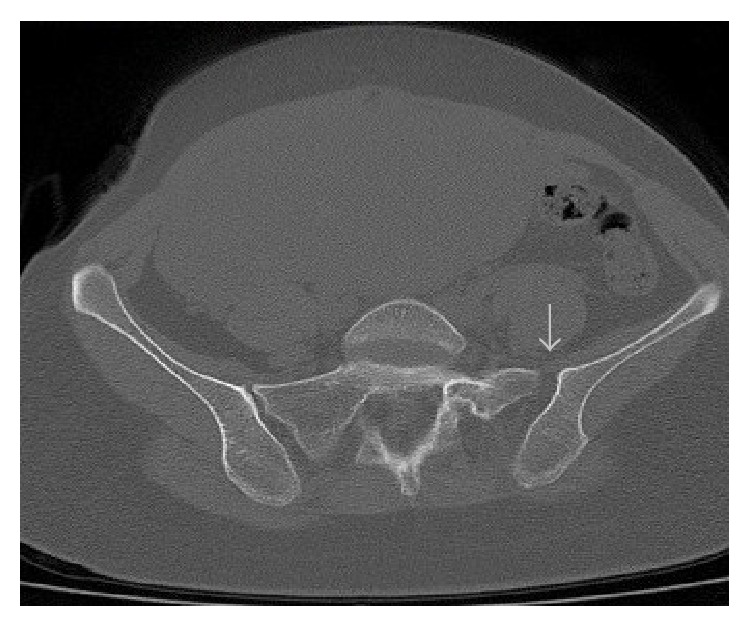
Computed tomography images demonstrating widening of the left anterior sacroiliac joint.
